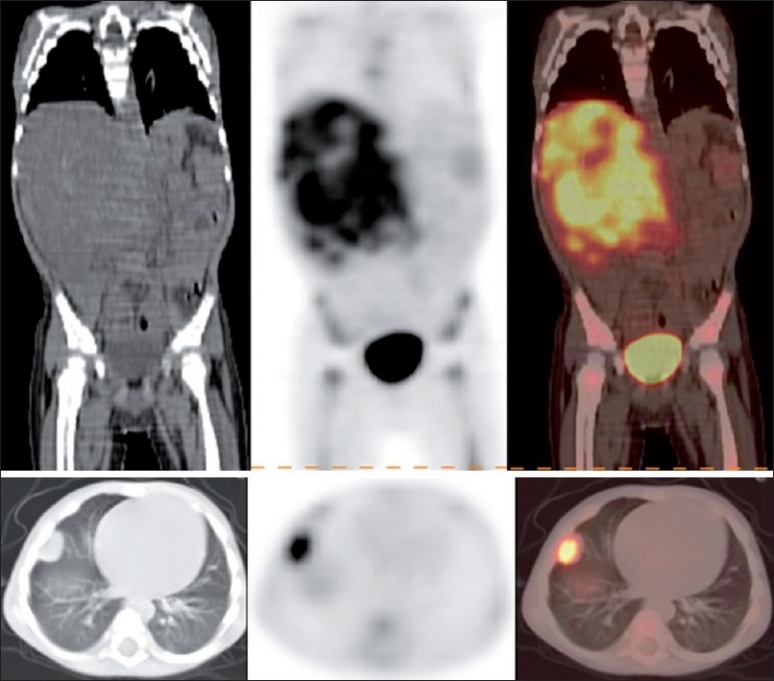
CT, PET and PET-CT images (L-R) showing the right suprarenal mass occupying right side of abdomen, pushing the liver superiorly and diffuse marrow metastasis (upper row). Transaxial CT, PET and PET-CT images in the same patient showing the presence of lung metastasis (lower row)
